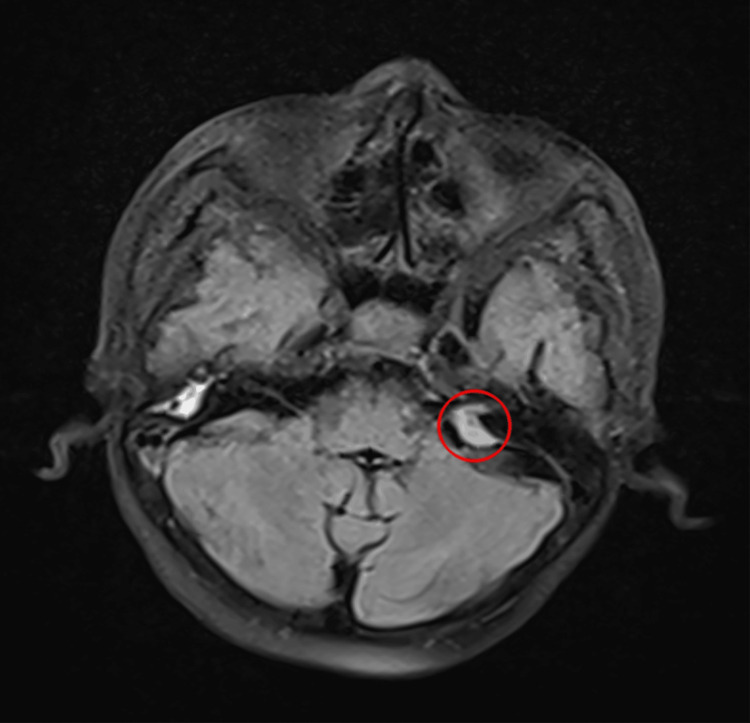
MRI gadolinium-enhanced T1-weighted axial image, showing a hypointense lesion (within the red circle) of 1.2 x 0.9 x 0.9 cm in the left CP angle extending into the left internal acoustic canal MRI: magnetic resonance imaging; CP: cerebellopontine
